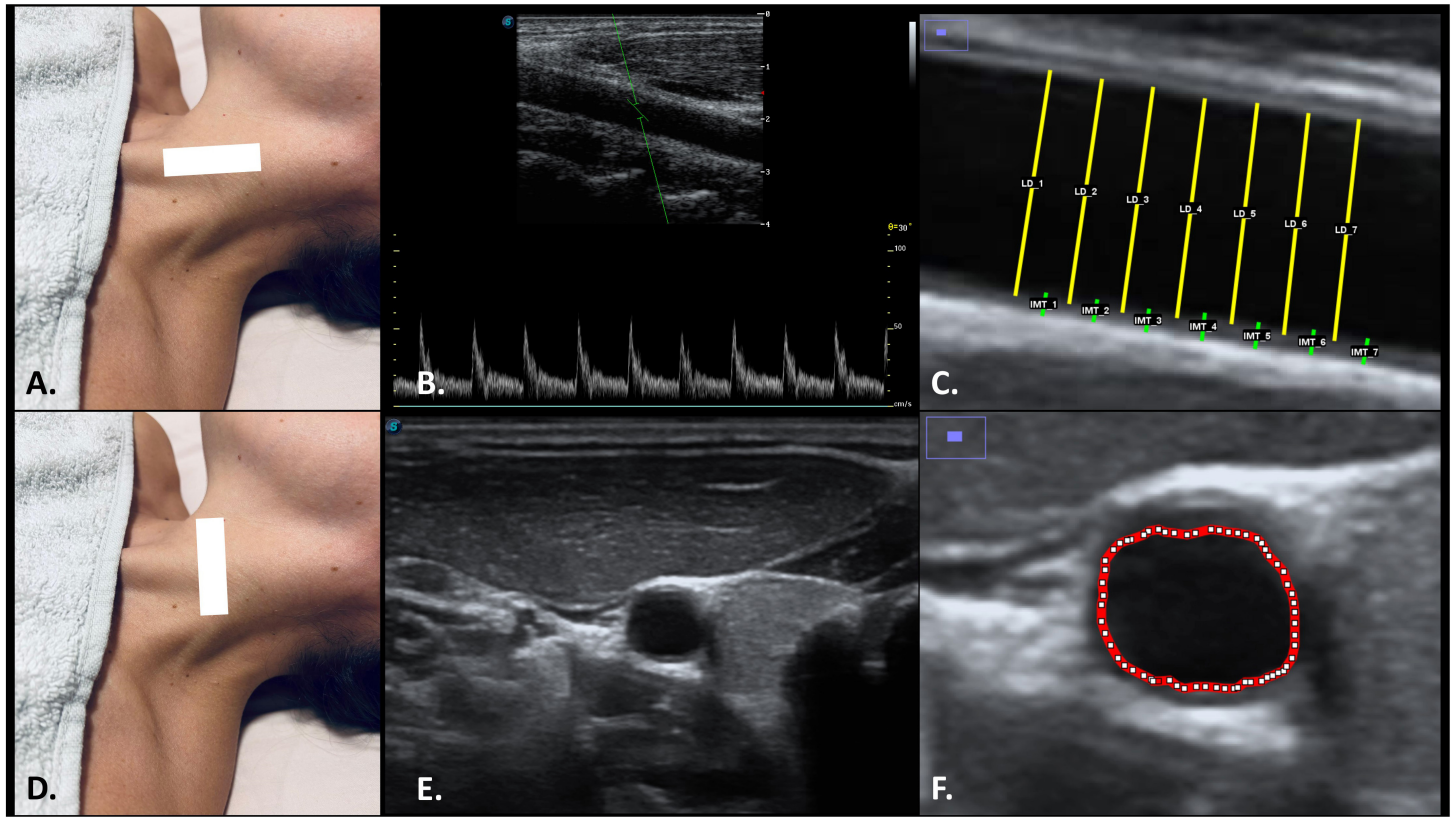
Ultrasonographic and ImageJ assessment of left CCA in long axis view (A) for PSV (B), LD and IMT (C); as well as in short axis (D) view for CSA (E, F). The probe was placed at the level of the left CCA using the SCM muscle as an acoustic window (A, B). Longitudinal sections on the left CCA (B, C) allowed to determine the peak systolic velocities (PSV) and the changes in the LD and IMT, while the transverse sections (E, F) allowed to determine the CSA. Abbreviations: CCA, common carotid artery; CSA, cross-sectional área; IMT, intima–media thickness; LD, lumen diameter; PSV, peak systolic velocities; SCM, sternocleidomastoid muscle.
